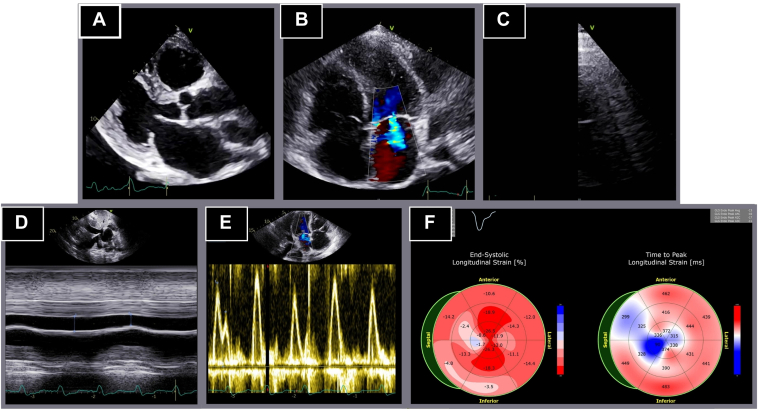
Transthoracic echocardiogram. Left ventricular ejection fraction of 44%. A: Parasternal long axis view. B: Apical 4-chamber view. C: B lines on pleural ultrasound. D: Severely dilated inferior vena cava (26 mm, with an inspiratory collapse 21%). E: Atrioventricular dissociation in transmitral inflow. F: Altered longitudinal strain of −11.1%.
